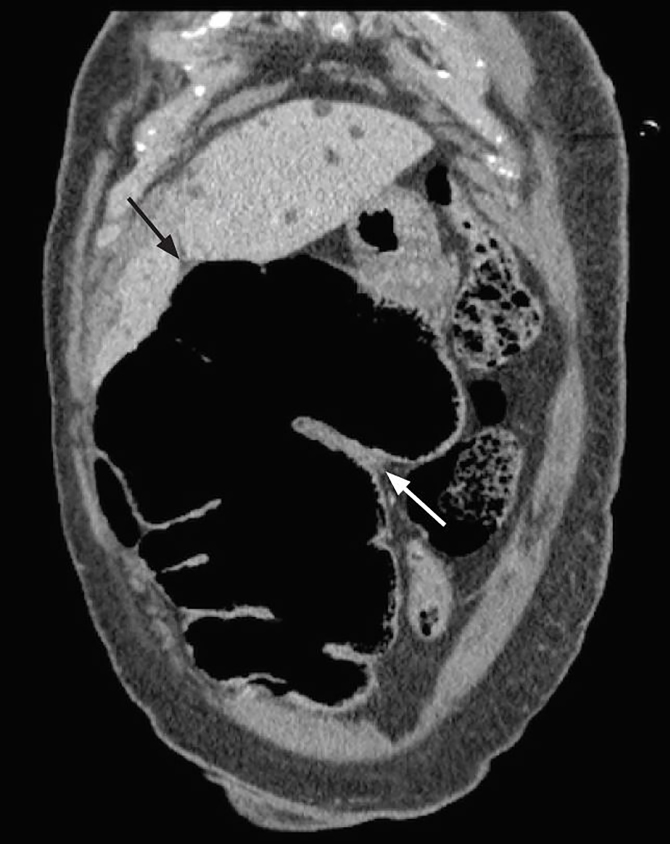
Computed tomography coronal image demonstrating marked gaseous distension of the distal cecum, which is flipped cephalad extending into the mid to right upper quadrant (black arrow). Comma sign (white arrow) refers to comma-shaped thickening of the root of the sigmoid mesocolon.
